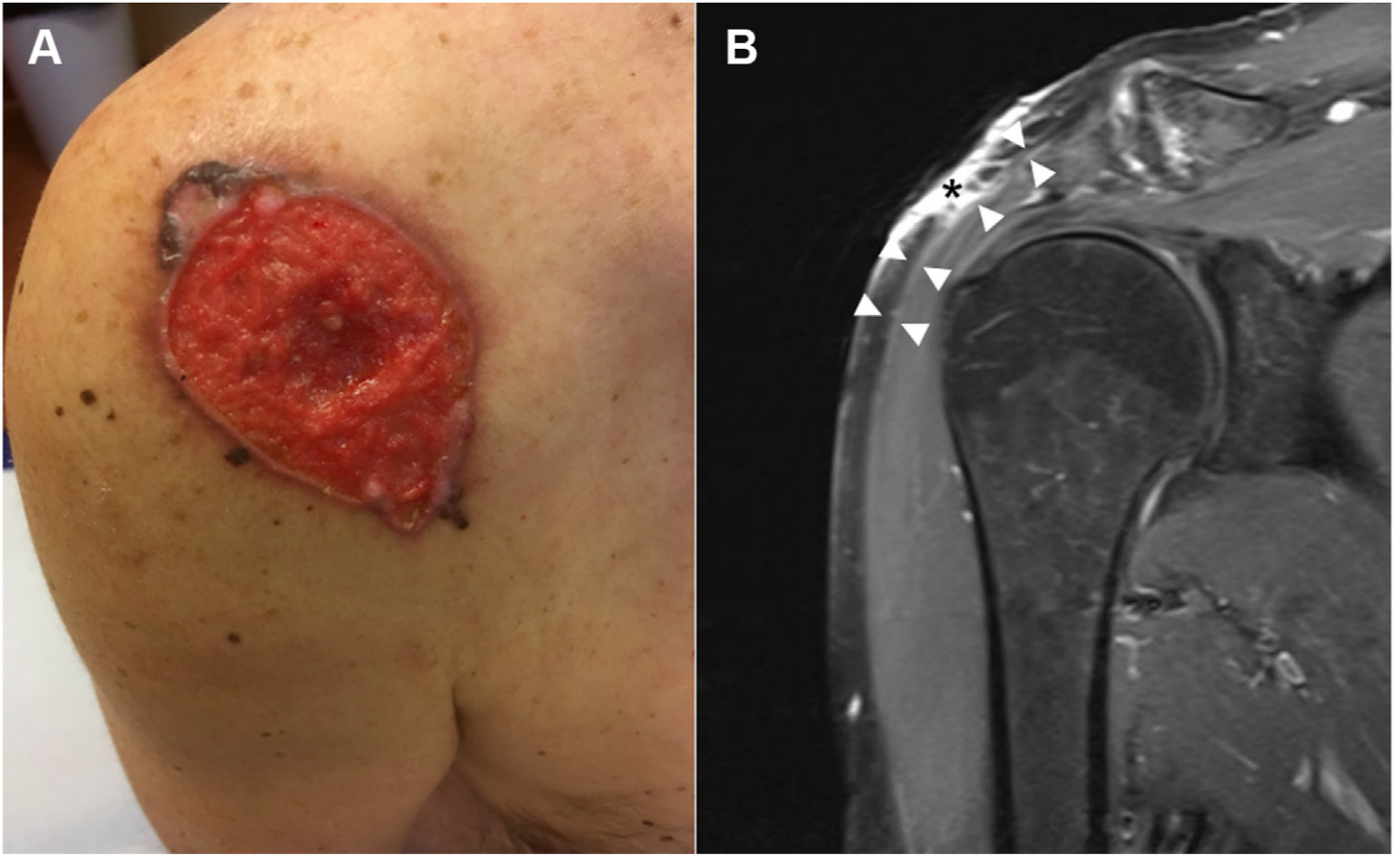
A, Clinical picture of a giant basal cell carcinoma (BCC) of the right shoulder. B, Sagittal view of a contrast-enhanced T1-weighted magnetic resonance imaging of the right shoulder, showing contrast enhancement in the BCC (*black asterisk*) and in the fascia of the deltoid muscle (delineated by *white arrowheads*).
